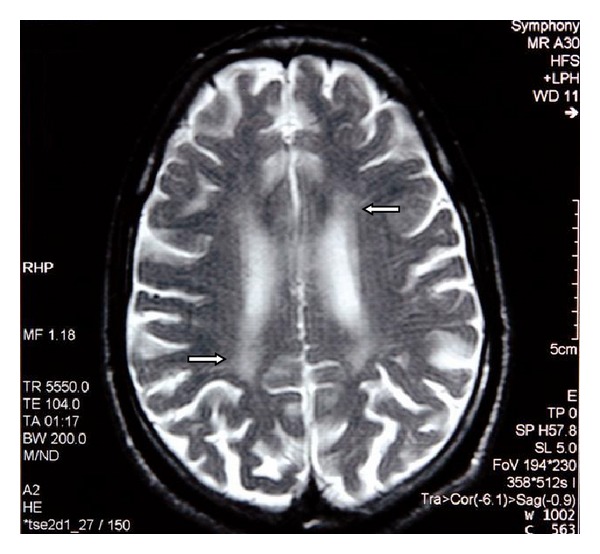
Axial T2 weighted MR image of the brain shows generalized cerebral atrophic changes with associated prominence of cortical sulci. There are high signal intensity changes in the periventricular white matter (arrows) along with some loss of grey matter-white matter differentiation.
